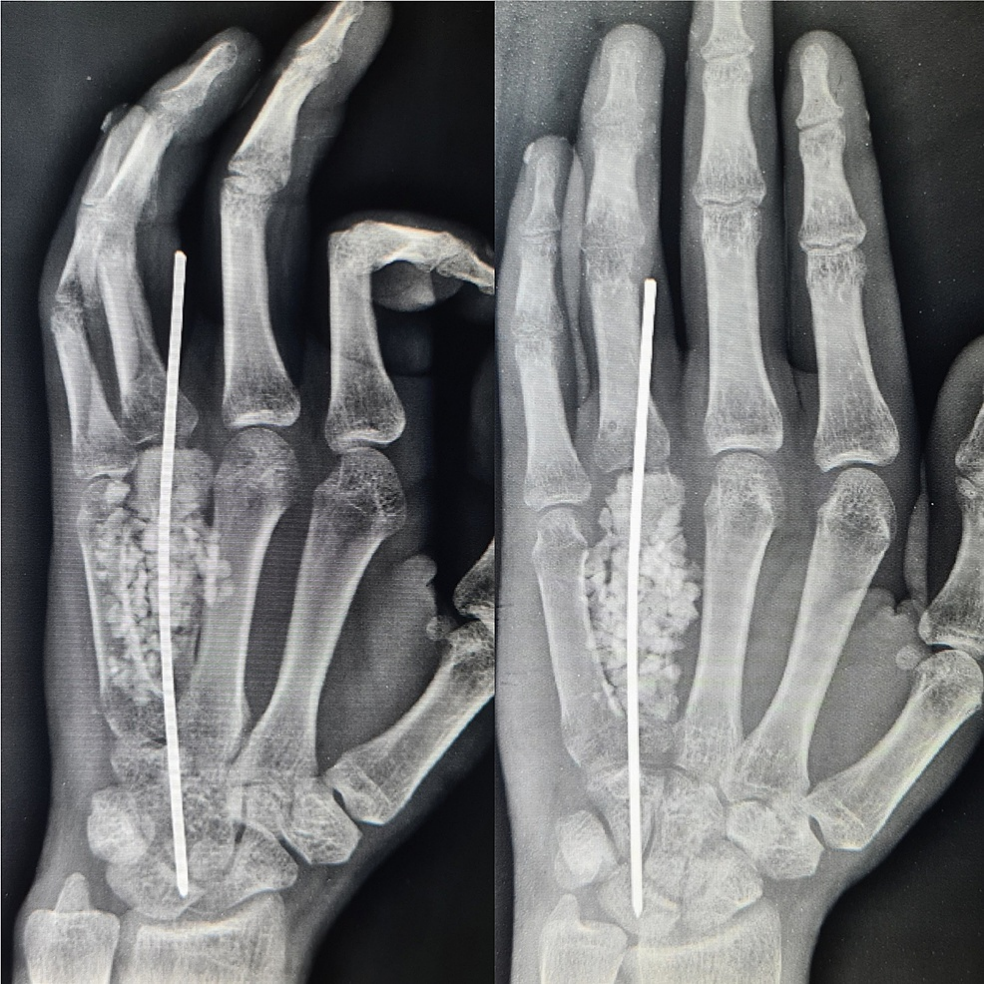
Post-op radiograph showing the intramedullary Kirschner-wire following tumor excision and augmentation of defect with bone graft.
